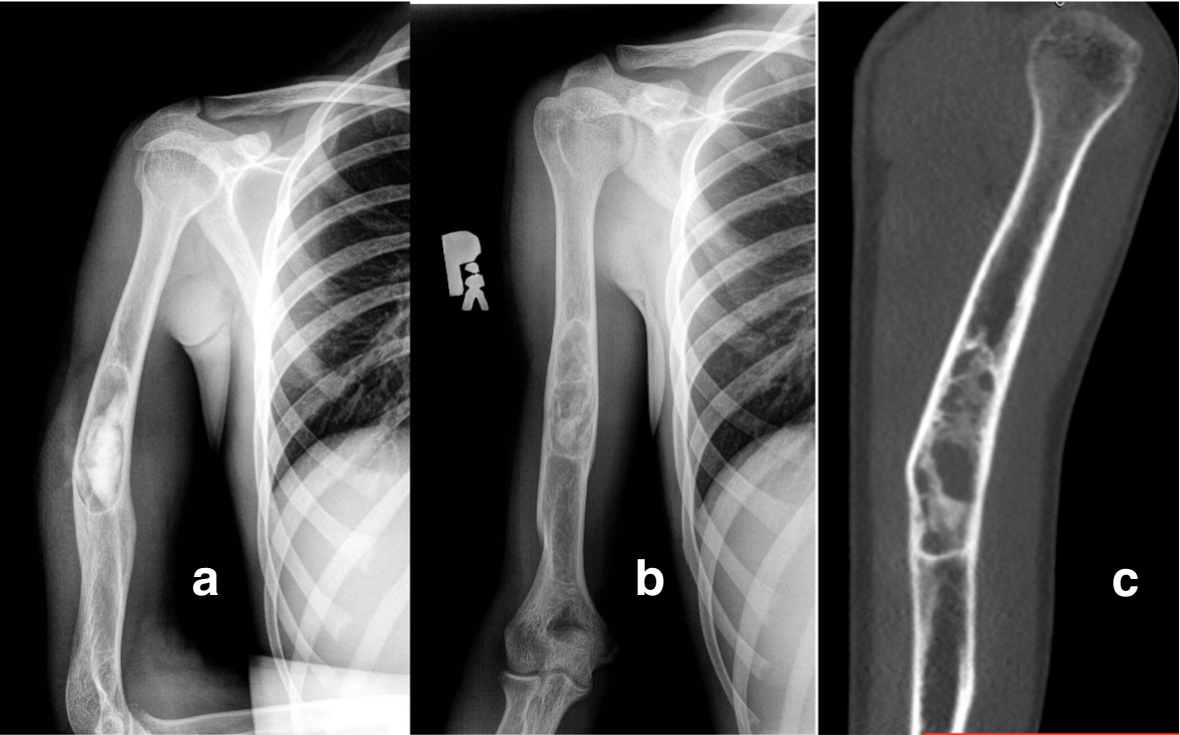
Twelve months follow-up with CT scan. Patient treated with 18 mL of CERAMENT™ due to a post-traumatic bone cyst. X-ray immediately post-op. a and after 12 (b) months. CT scan after 12 months demonstrating trabecular bone bridging (c)
